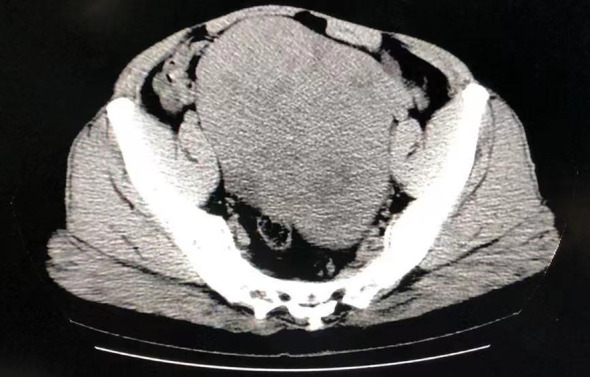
Computed tomography obtained showing space-occupying lesions in the lower abdomen.
